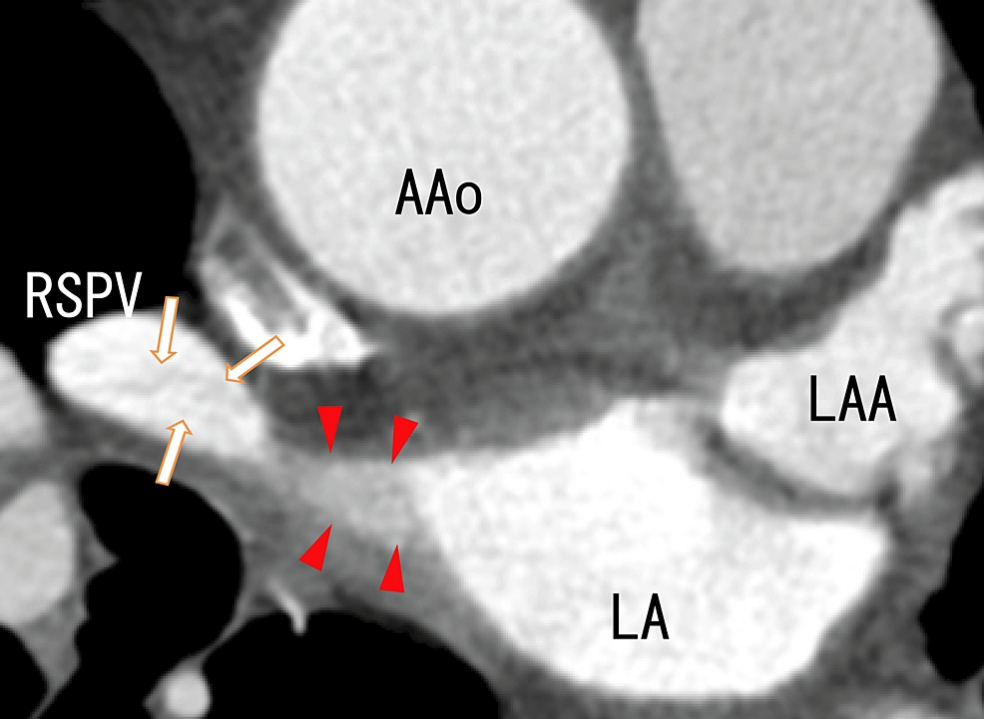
Axial images from an 80-MDCT scan showing thrombi in the RSPV and LA Axial images from an 80-slice multidetector computed tomography (80-MDCT) scan showing the right superior pulmonary vein (RSPV) and left atrium (LA). There were rather dark areas in the RSPV (arrows), suggesting that there were thrombi in the RSPV. There were dark areas in the left atrium (arrowheads), suggesting that there were thrombi in the left atrium. No left atrium diverticula (LADs) were detected. AAo, ascending aorta; LAA, left atrium appendage
